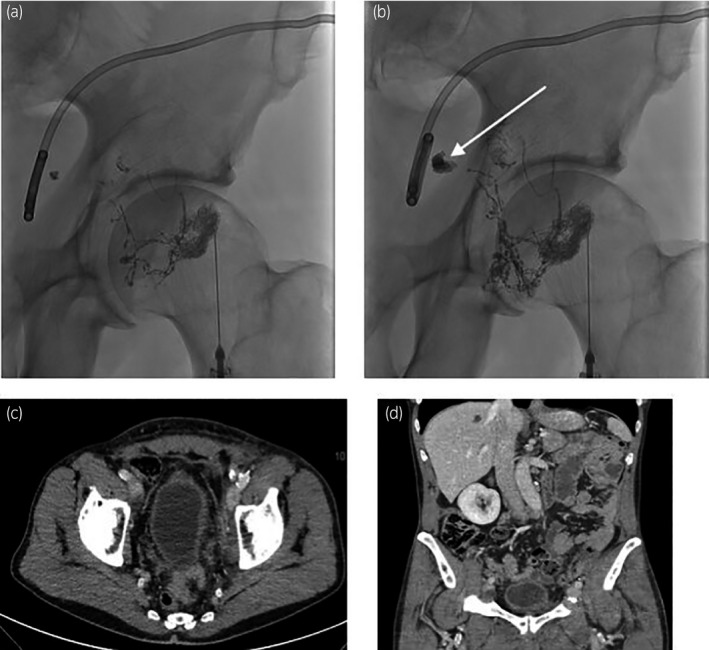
Patient 2: lymphangiography and follow‐up. (a) Left inguinal lymphangiography. (b) Later images showing a leakage from external iliac lymph ducts next to the percutaneous catheter, followed by a glue injection into the closest lymph node. (c, d) CT scan 6 weeks after the left lymphatic embolization. No residual lymphocele.
